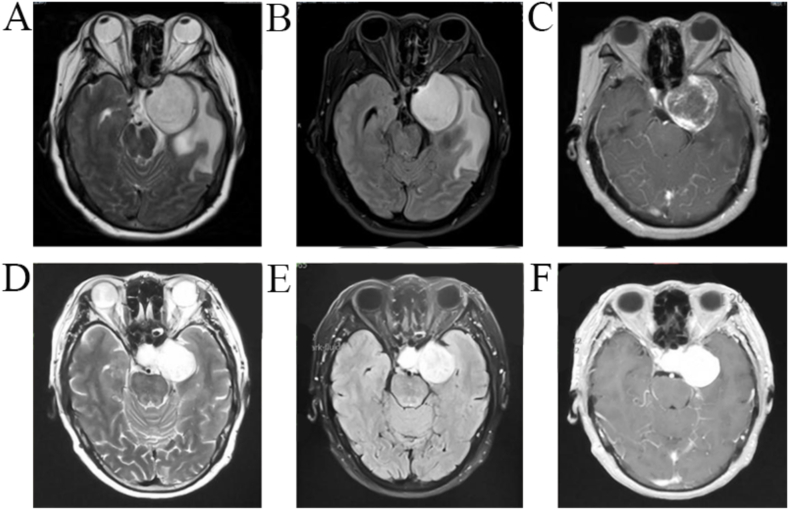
Imaging characteristics of Type A (A, B and C) and Type B (mulberry type) (D, E and F) CSH, revealing significant cavernous sinus swelling and extrusion sign without dural tail sign. (A) Homogenous hyperintensity on T2WI. (B) Hyperintensity on FLAIR. (C) Heterogenous enhancement of enhanced T1 (D) Homogenous marked hyperintensity on T2WI close to the cerebrospinal fluid's signal. (E) Homogenous hyperintensity on FLAIR. (F) Homogenous enhancement of enhanced T1.
